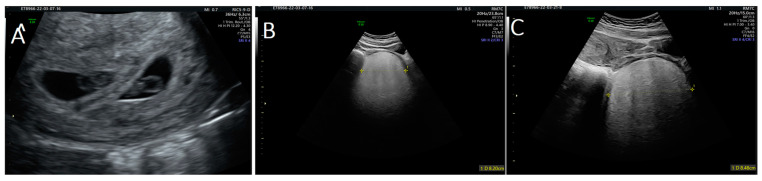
Ultrasound images of: (A) twin pregnancy; and (B,C) angiomyxoma.
